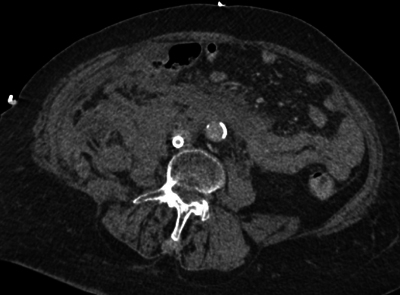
An axial CT of extensive intra- and retroperitoneal blood at the level of the infrarenal aorta.
